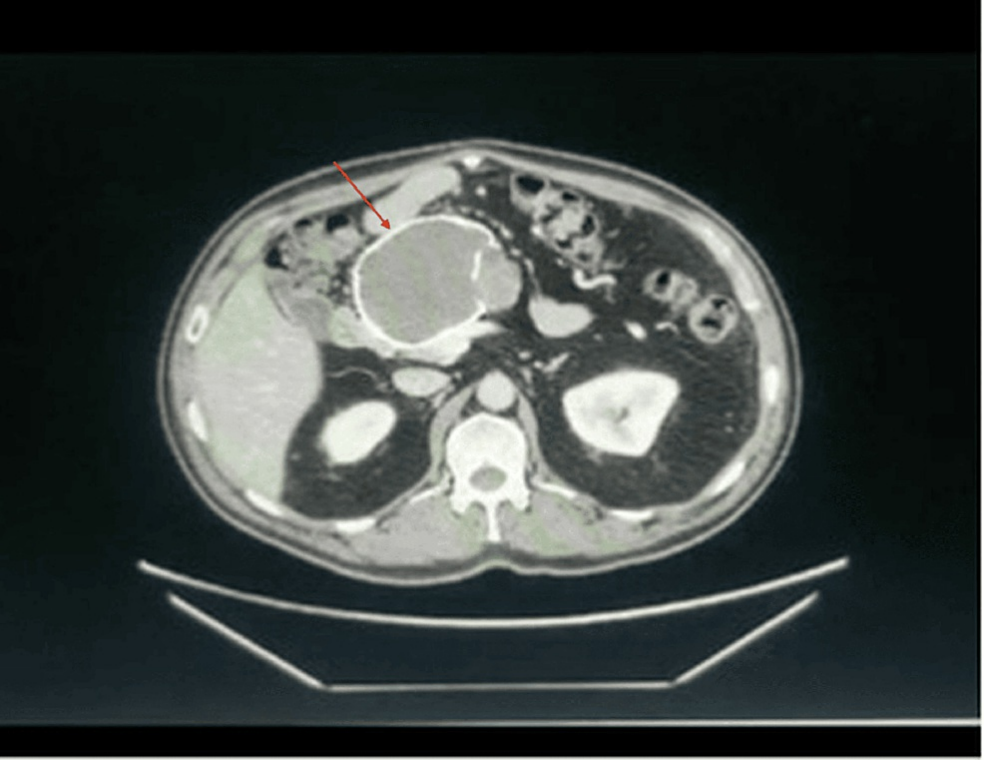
Contrast-enhanced CT scan of the abdomen from 2023. The image reveals a large (9 cm) non-enhancing septated lesion in the head of the pancreas, featuring hyperdense internal material, a thick rim, and coarse calcifications within the septa. Notable atrophy of the pancreatic parenchyma is also observed. There has been no significant change in the size of the lesion compared to the prior exam in 2019 and the initial imaging in 2006.
